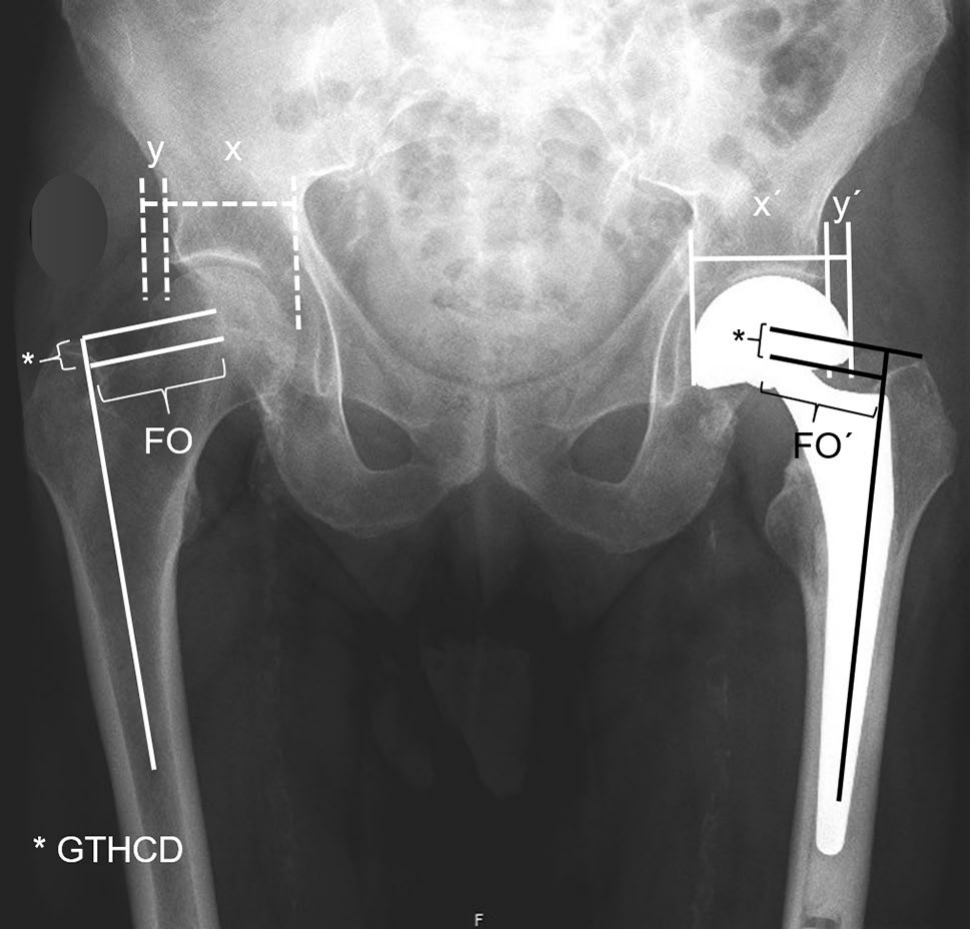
Standard pelvic radiograph with delineated lateral femoral offset of the unaffected side (FO) and after hemiarthroplasty (FO´), femoral head extrusion index [FHEI = *x*/(*x* + *y*)], bipolar head extrusion index [BHEI = *x*´/(*x*´ + *y*´)], greater trochanteric tip hip center distance (GTHCD)
